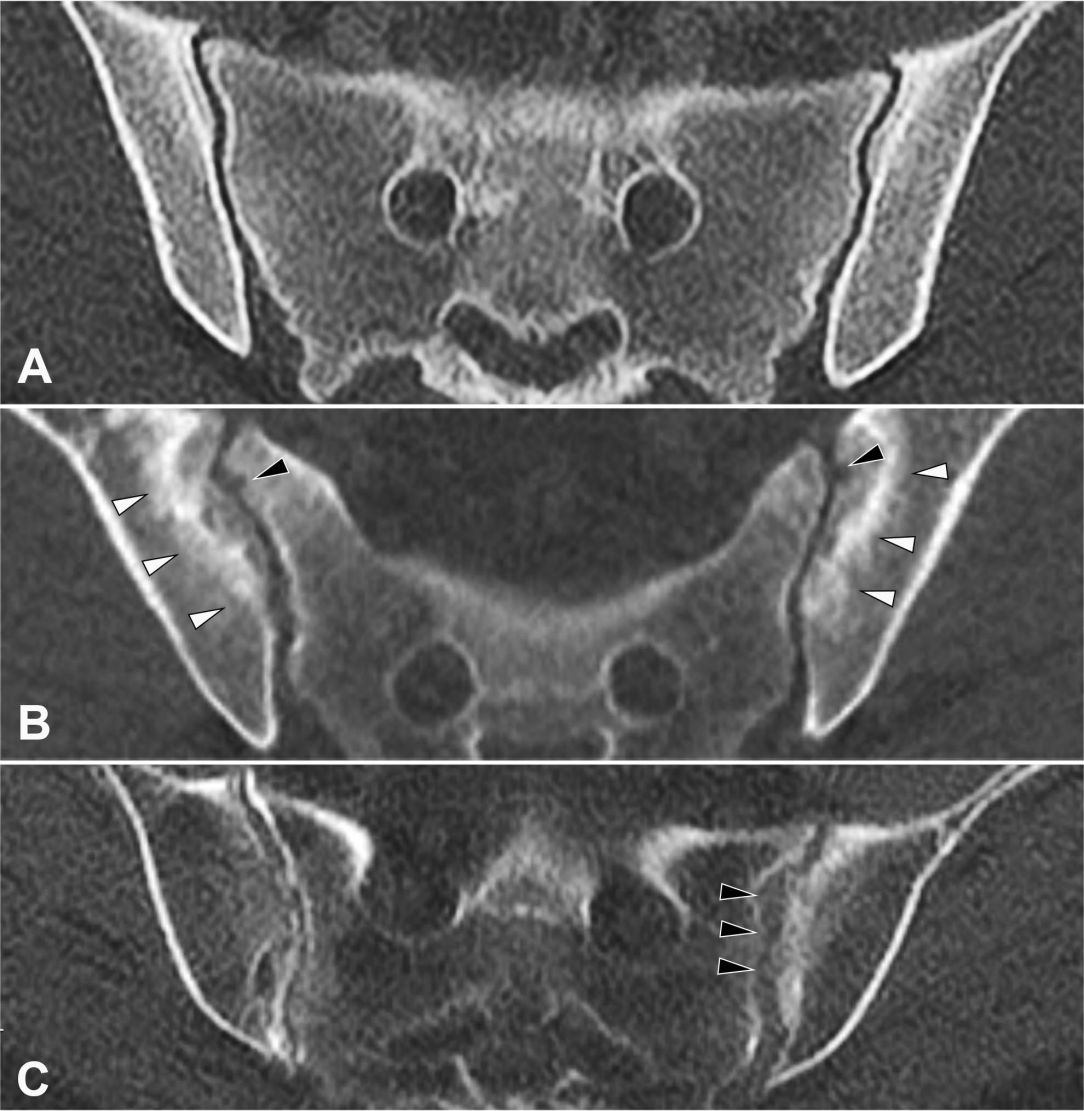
Example of joint alterations in sHPT. Axially reconstructed high-resolution CT images. (A) Healthy control patient with normal SIJ. (B) Patient with secondary hyperparathyroidism: note the subchondral bone resorption mimicking erosions (black arrowheads) as well as the sclerotic rim around the resorption zone (white arrowheads). (B) Patient with secondary hyperparathyroidism: note the irregular, pseudo-widened left joint space (black arrowheads) compared to the regular joint space on the right.
